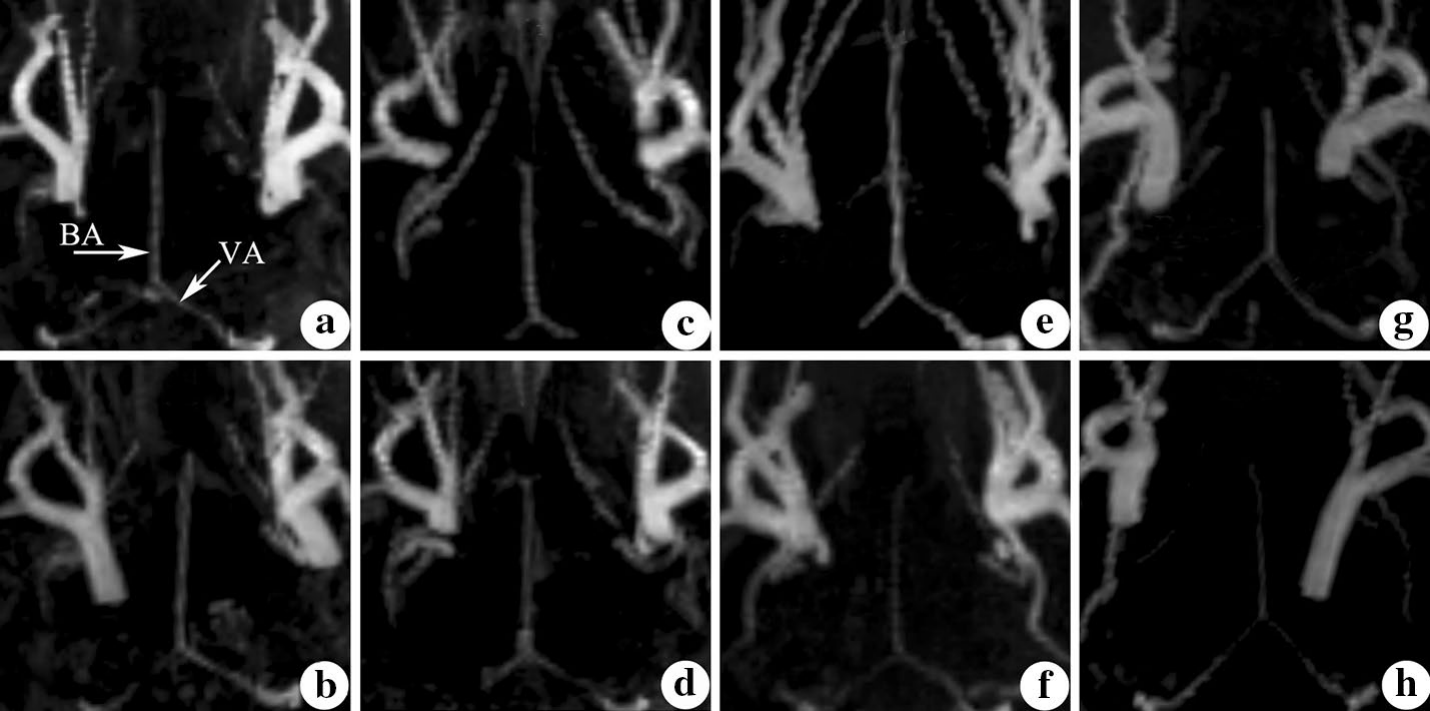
Observation of basilar artery in MRA before and after modeling. a, b Manual control group. c, d Ultrasound guided control group. e, f Manual model group. g, h ultrasound guided model group. a, c, e, g Before modeling. b, d, f, h 5 days after modeling. *BA* basilar artery, *VA* vertebral artery
